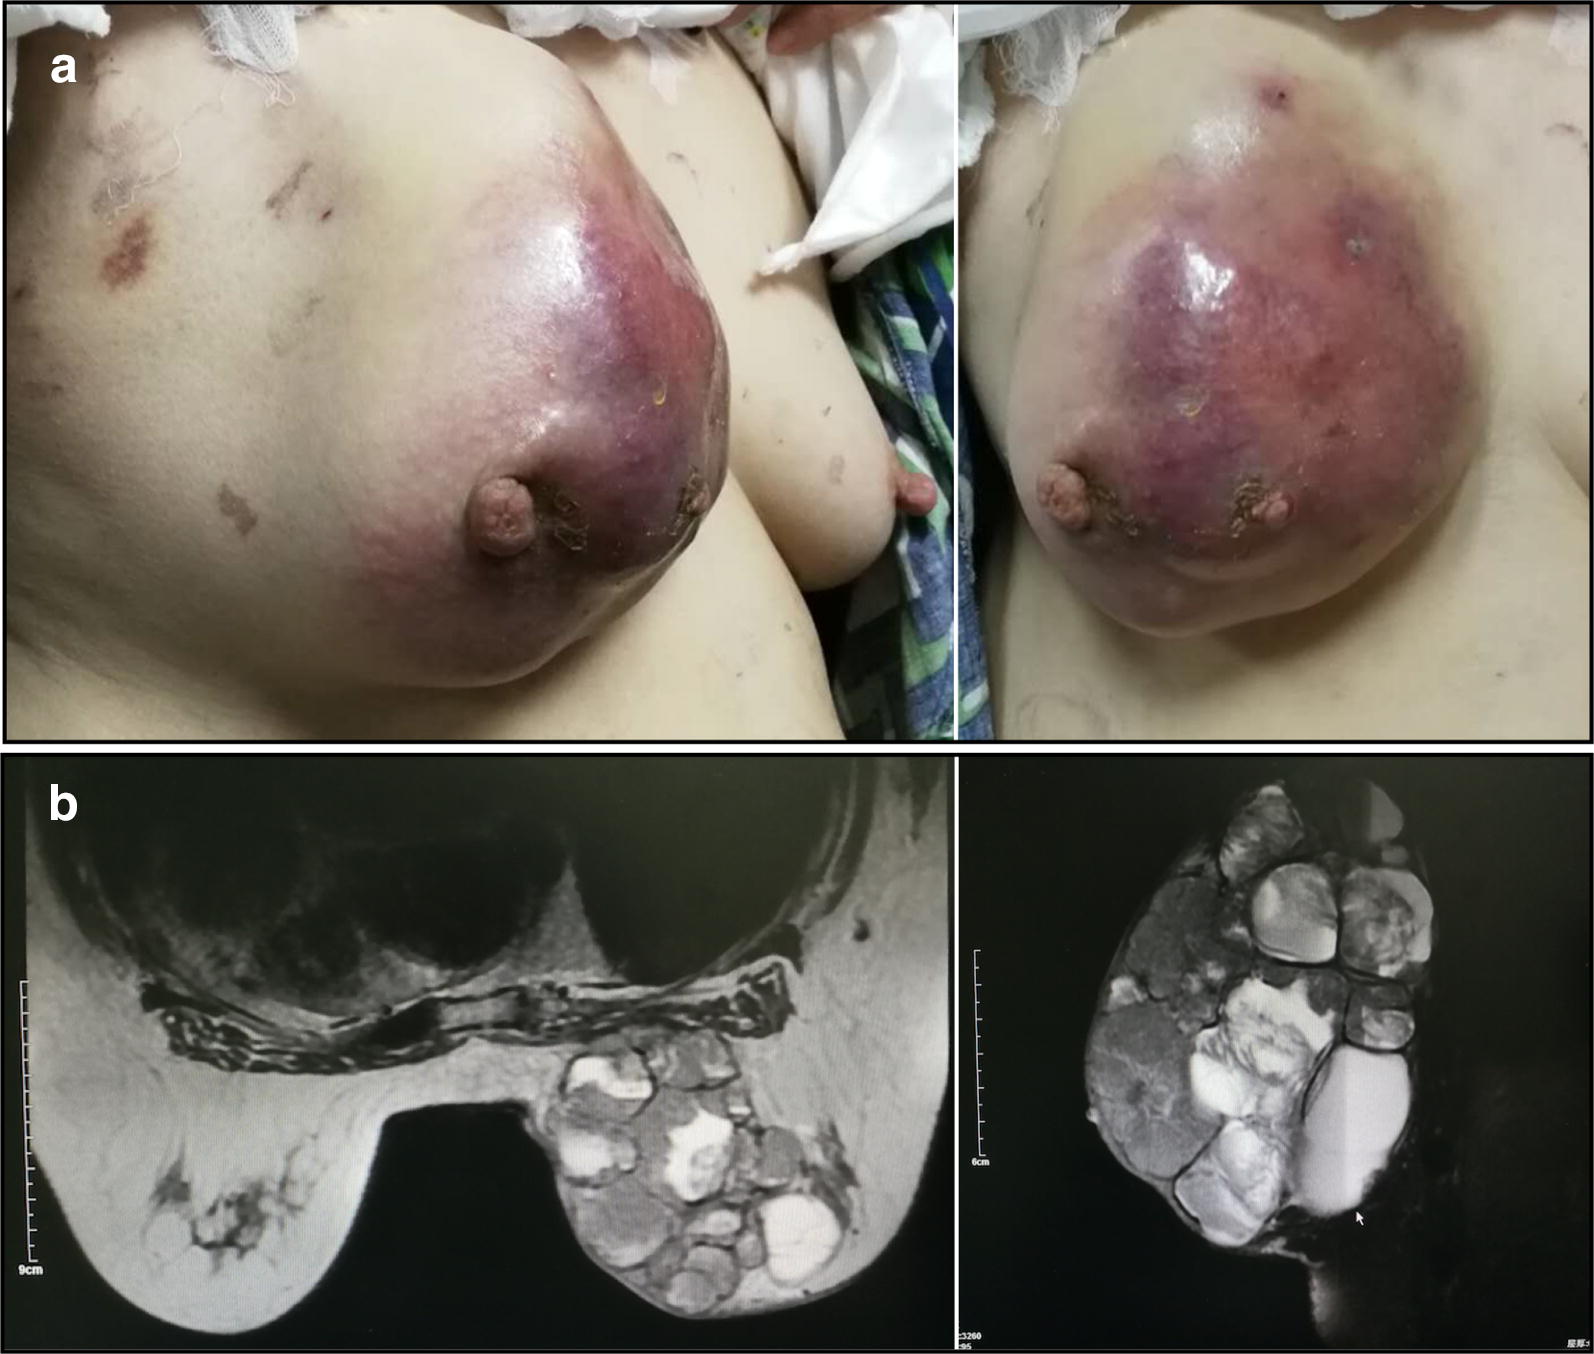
A giant papillary carcinoma of right breast in a 63-year-old Chinese woman was observed by visual examination and MRI scan. a The tumor increased rapidly 1 month ago, the skin was red and swollen, and it could touch the size of 12*12 cm hard mass with skin ulceration. b Chest MRI showed large cystic and solid mass of irregular shape. The solid part was significantly enhanced after enhancement
